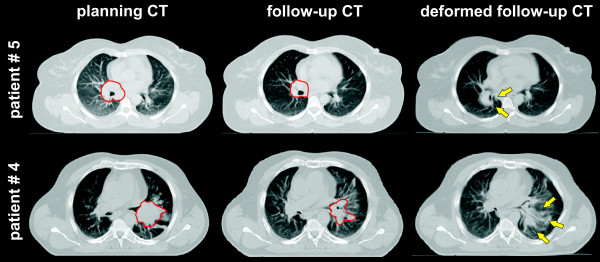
Suboptimal results of deformable image registration. The contours of the macroscopic primary tumor in the planning CT and the follow-up CT are shown in red and arrows point to artefacts after deformable image registration. Patient #5: deformation artefacts of the hilar bronchi (which were included into the GTV). The rather large amount of normal tissue within the GTV is probably responsible for this poor performance of DR. Patient #4: deformation artefacts after tumor shrinkage with a decay of the GTV.
